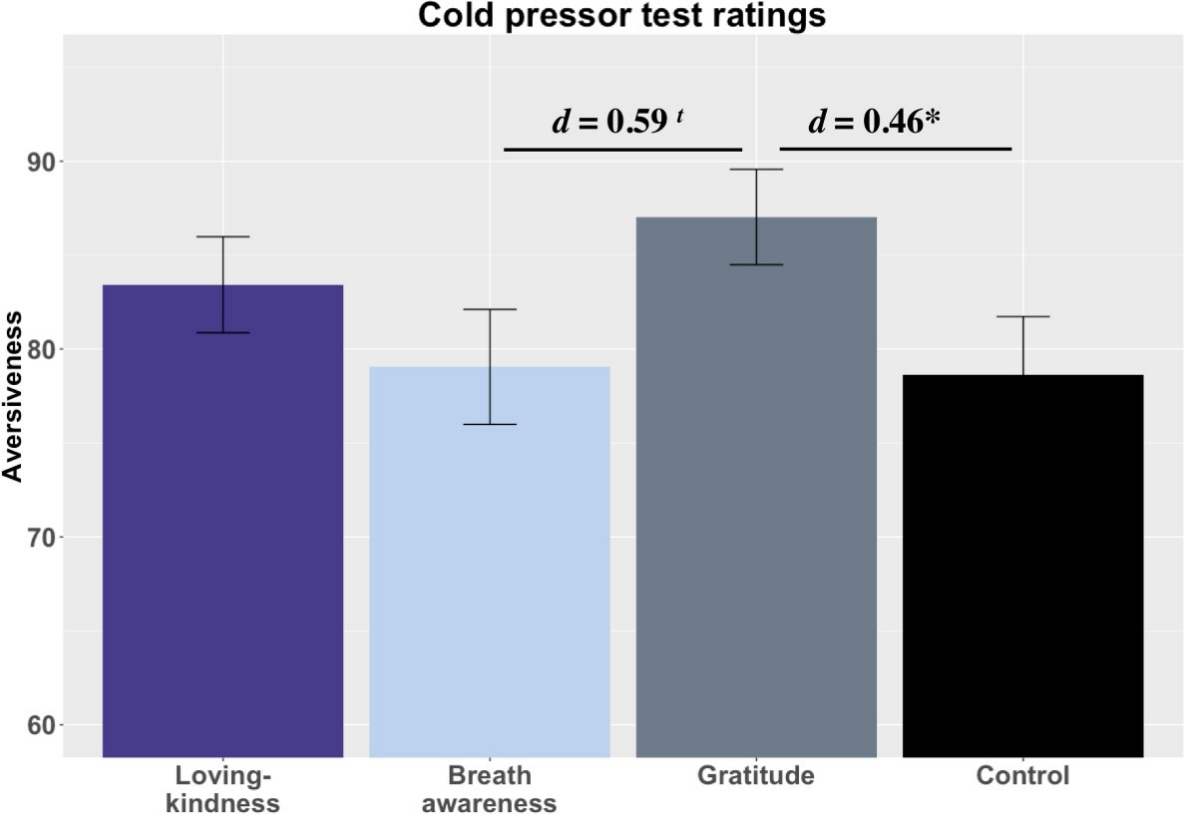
Cold pressor aversiveness ratings. Notes: * = *p* < .001, * = p* < .01,  = p <* .05, *t = p* < .10; 4A. Loving-kindness = LK; Breath awareness = BA; Gratitude = GT; Control = CT.
